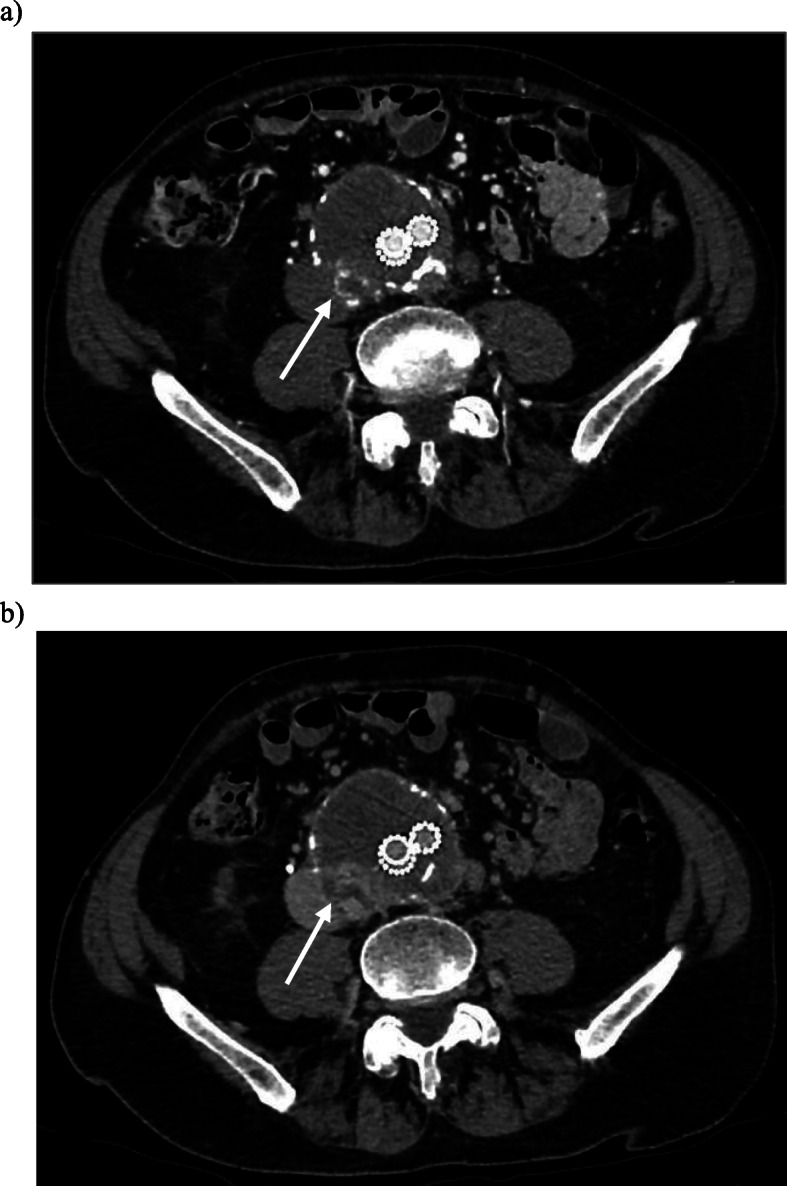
Axial arterial phase CT images of the abdominal aorta demonstrate pooling of contrast (arrow) on the arterial phase (a) with increase pooling on the delayed phase (b)
